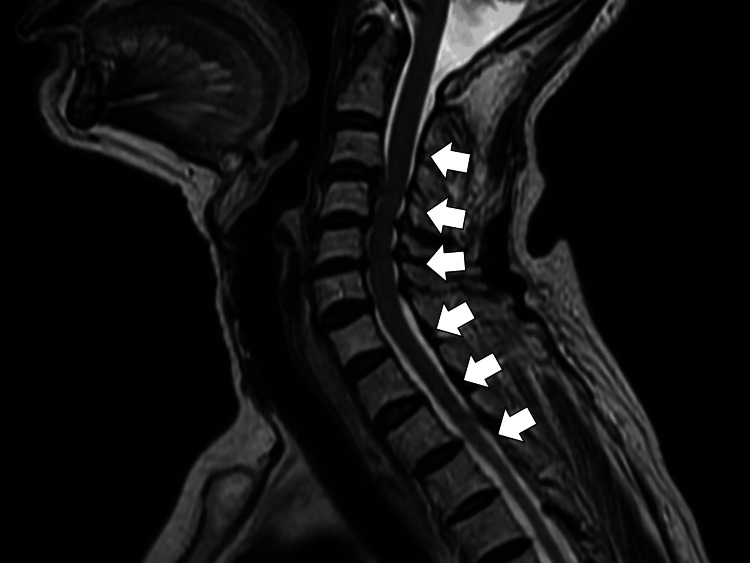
Cervical spinal magnetic resonance imaging (MRI) T2-weighted images of the cervical spine did not demonstrate any such abnormal signal intensity in the posterior columns, at the C3-C5 levels.
